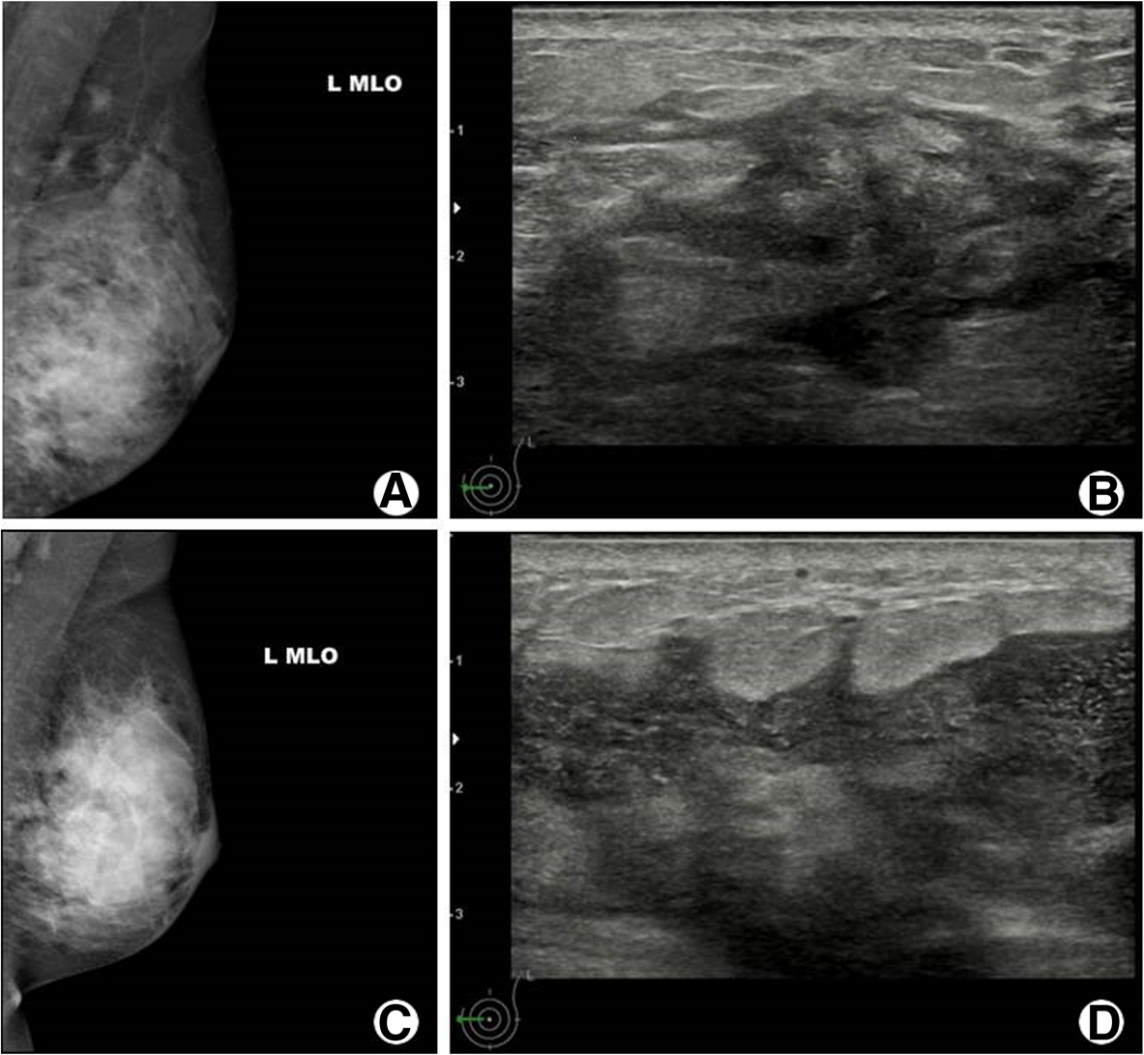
Findings of mammography and ultrasonography of two patients with granulomatous lobular mastitis. Patient 1. There was asymmetric increased density on *left lower* breast (a) and ultrasonography reveals a hypoechoic and irregular shaped mass with ill defined margins (b). Patient 2. Oblique view of *left* breast demonstrated diffusely increased asymmetric density on *left* breast and enlarged axillary lymph nodes (c). Ultrasonography showed irregular hypoechoic mass with internal debris with skin thickening, consistent with abscess (d)
